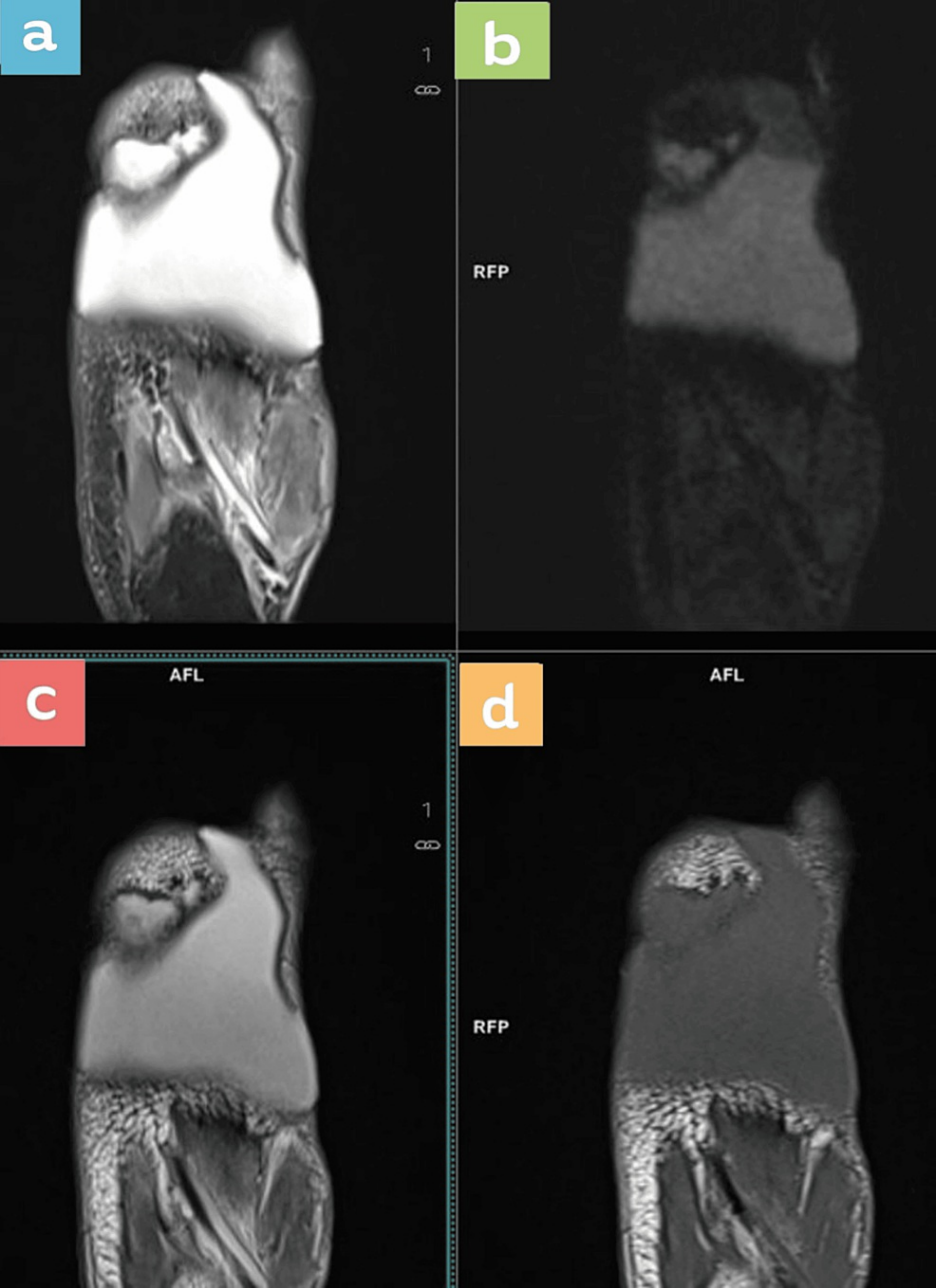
MRI axial sections at the level of collection showing the serous fluid collection Top row (left to right): a) proton density fat saturated (PDFS), b) short tau inversion recovery (STIR). Bottom row (left to right): c) T2, d) T1.
